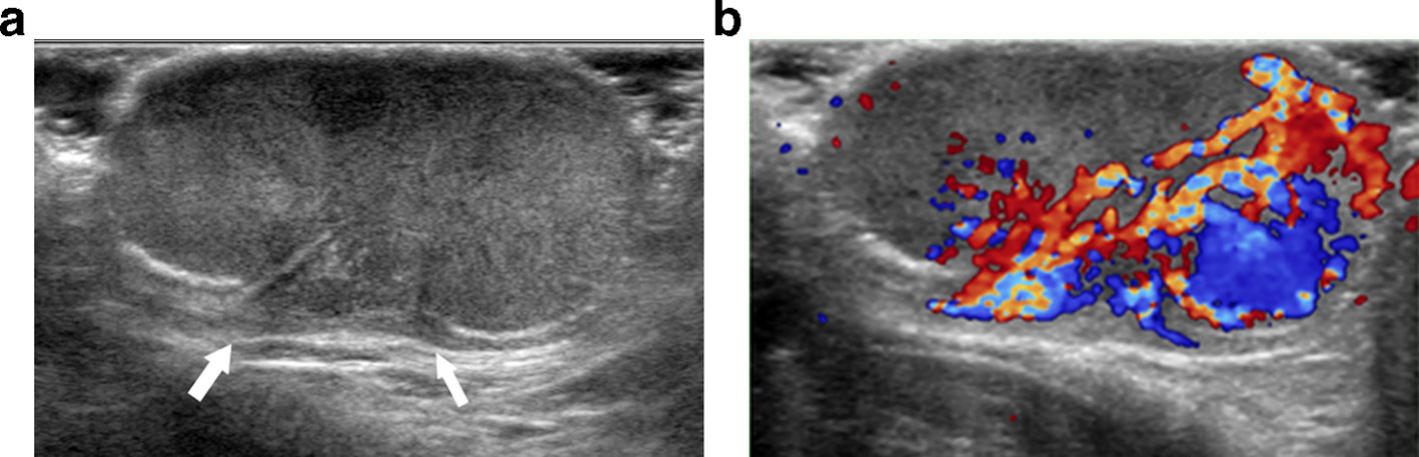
A, Ultrasonography shows a mild heterogeneous hyperechoic polypoid mass in the right upper scrotal wall. The stalk of the mass is connected to the dermis, but the subcutaneous fat is not invaded (white arrows). B, Color Doppler ultrasonography shows remarkable intratumoral vascularity in the peripheral portion of the mass from the stalk.
